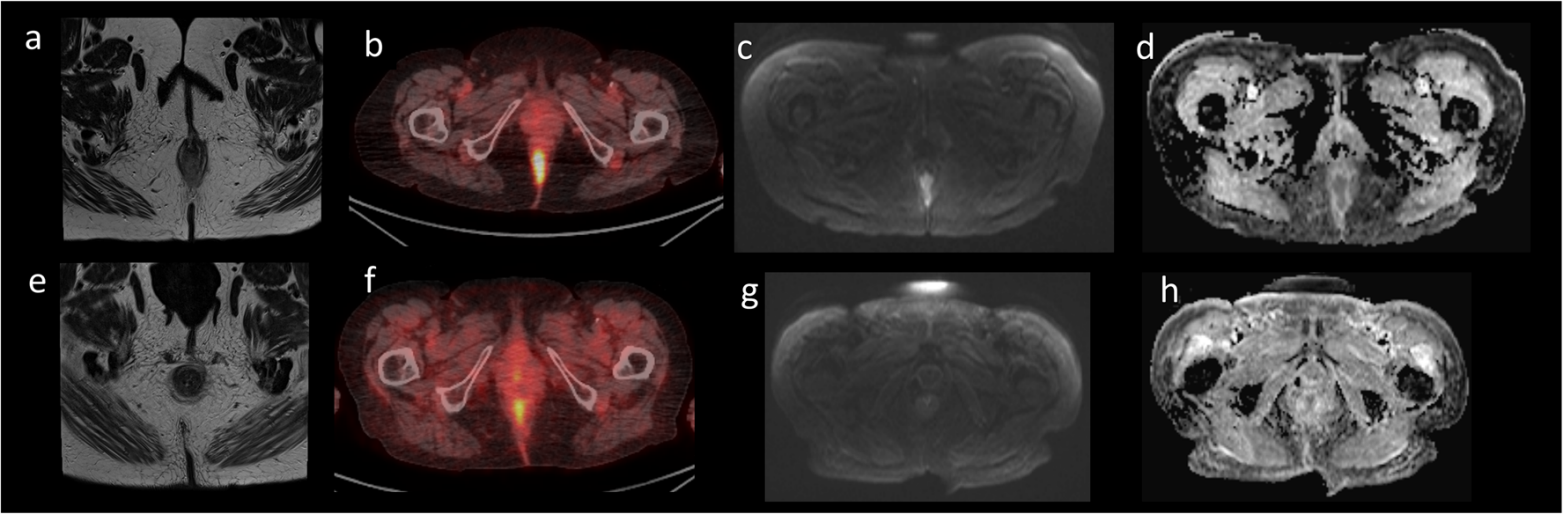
84-year-old female. Axial images. MRI response assessment: complete, PET-CT response assessment: partial, combined response assessment: complete. a Pre-treatment T2W MRI demonstrates tumour involvement between the 4 o’clock and 6 o’clock position. b Pre-treatment PET-CT demonstrates avid FDG uptake within the anal canal. c DWI demonstrated diffusion restriction (high signal). d The ADC confirms true restriction (low signal). e Post-treatment T2W MRI demonstrates replacement of the primary tumour with a small area of fibrotic tissue. f Post-treatment PET-CT demonstrates residual focus of moderate FDG uptake which may either represent residual disease or post-treatment inflammatory change. g The DWI sequence does not demonstrate restriction. h The lack of diffusion restriction is confirmed by the ADC map. Overall, due to the lack of diffusion restriction and evidence of fibrotic tissue on the T2W MRI, the patient was considered as a complete responder. The focus of FDG uptake on the response assessment FDG PET-CT was most likely inflammatory in nature
